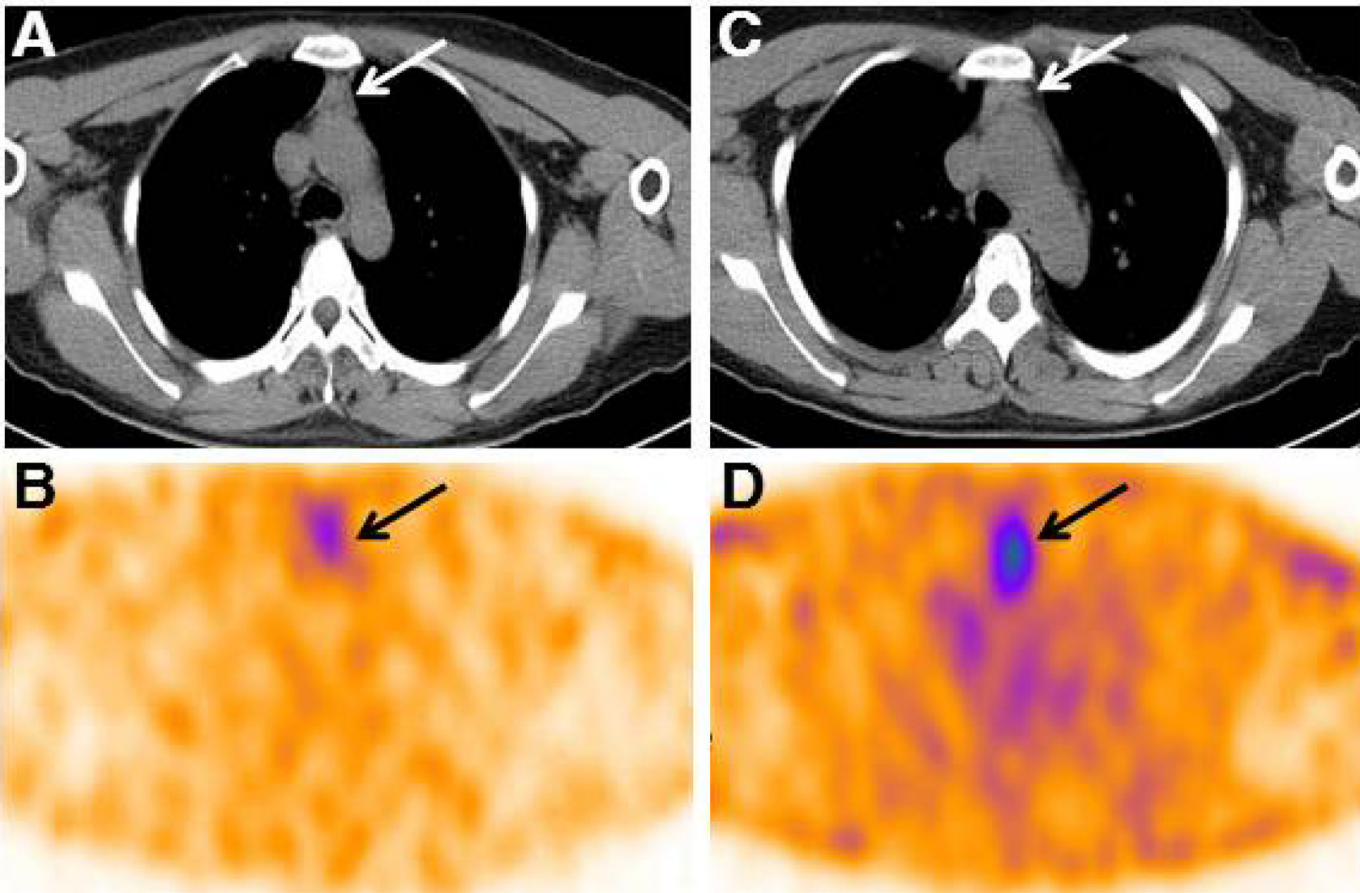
Two representative ^99m^Tc-3PRGD2 SPECT/CT images of TENIS patients with different serum Tg levels (A–B) Patient 9 with stimulated Tg of 18.2 ng/mL demonstrated a uptake ratio of 1.8 in thymus; (C–D), Patient 3 with stimulated Tg of 58.5 ng/mL showed a T/B ratio of 3.2 in thymus.
